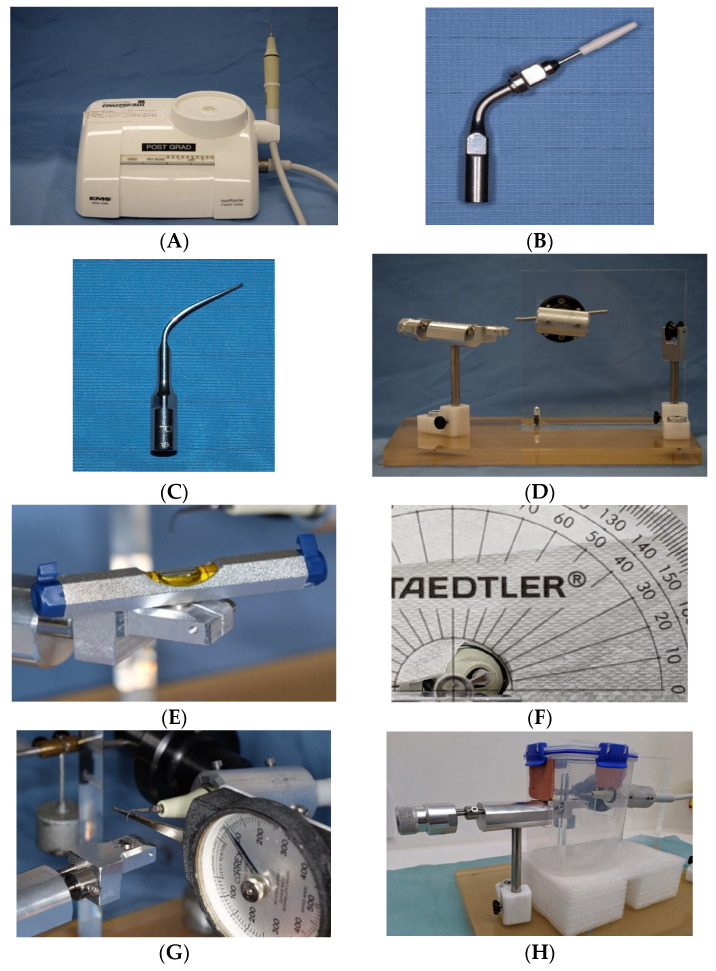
Setup of ultrasonic scaling with metal and PEEK tips: miniMaster piezon scaler E.M.S. (A), steel tip P, E.M.S. (B), PEEK Instrument PI, E.M.S. (C), custom instrumentation device (D), horizontal disc platform (E), scaling tip fixed at 15° (F), force controlled at 100 g (G), and enclosure for rinsing solution collection (H).
